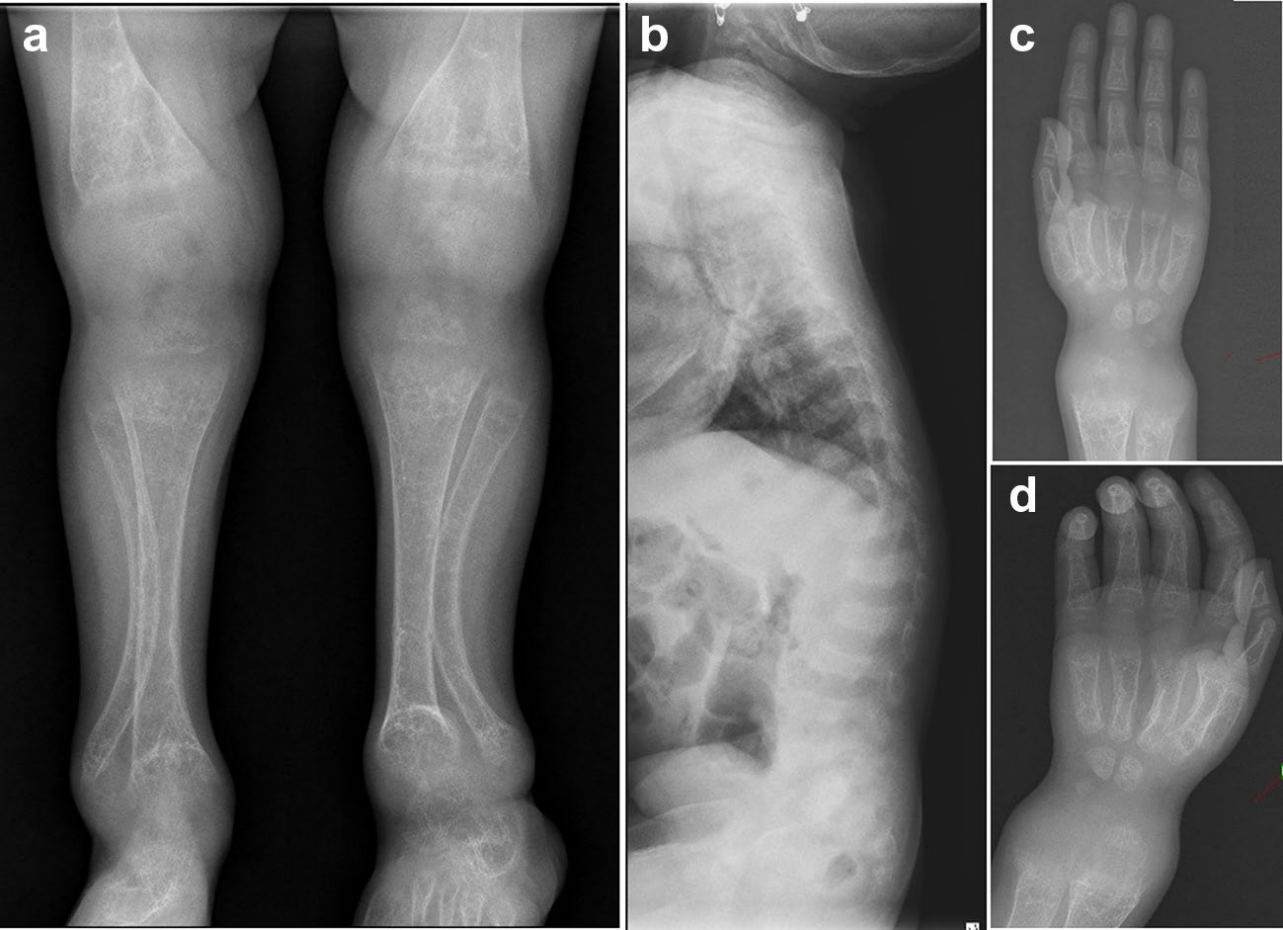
Radiographs of an individual with vitamin-D-dependent rickets type IIA. P5 (age 4 y) shows severe osteopenia, small epiphyses at the knee, irregular metaphyses, bending of the fibula (a), dorsal–lumbar kyphosis (b), and cupping of radius and ulna (c and d)
